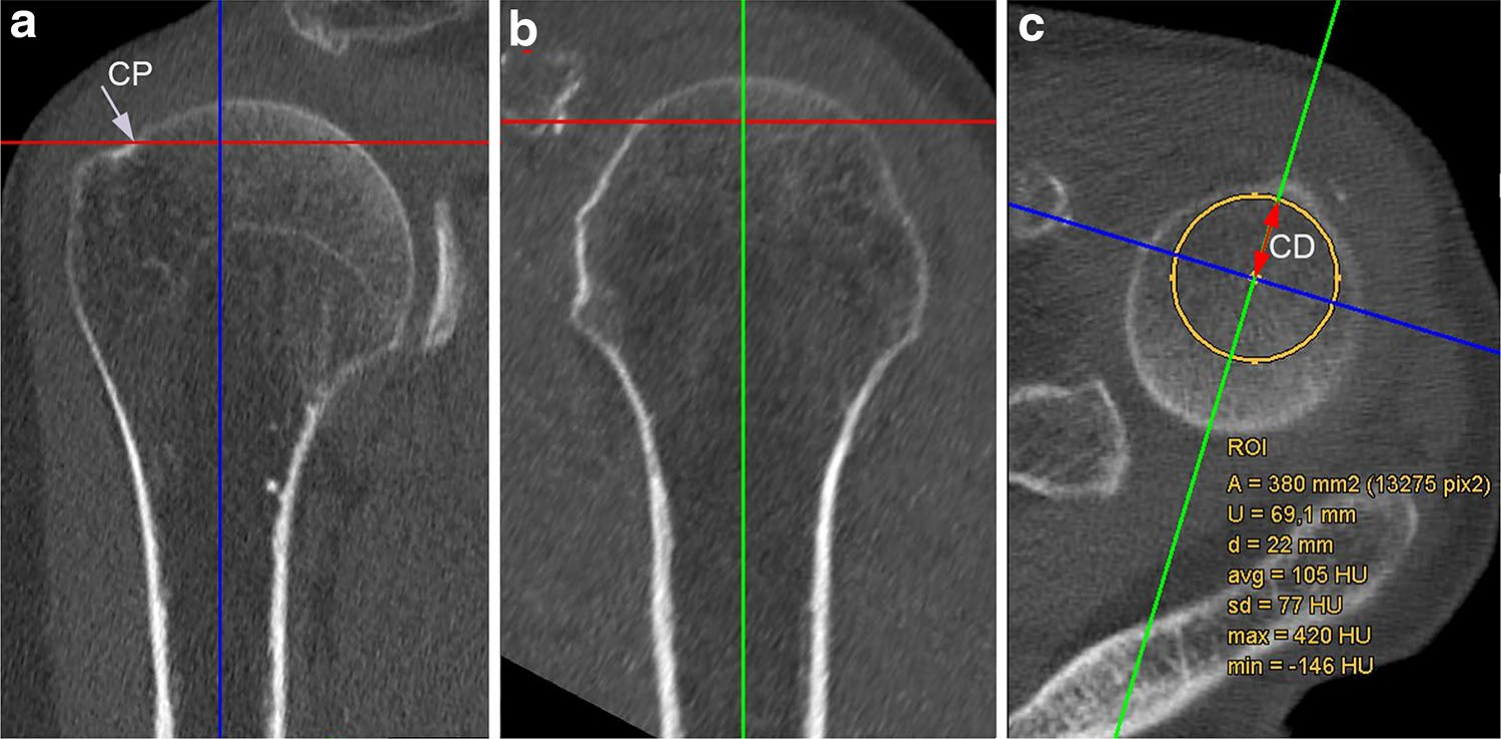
Multiplanar two-dimensional CT reformations of the proximal humerus in three perpendicular planes: true anteroposterior (a), true lateral (b), and axial (c). Critical distance (CD) was defined as the distance between the straight antegrade nail’s ideal entry point (indicated by the* vertical axis* through the center of the humeral medullary cavity extrapolated through the humeral head) and the critical point (CP), and was assessed in the axial reformation at the level of the CP (c) (see text for details)
